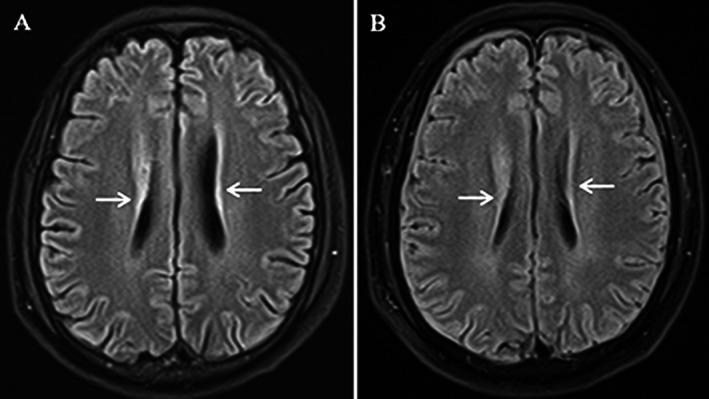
Brain MRI scans at symptom onset (A) and after symptom improvement (B) of Case 1. White arrows point to the patchy hyperintensity.
